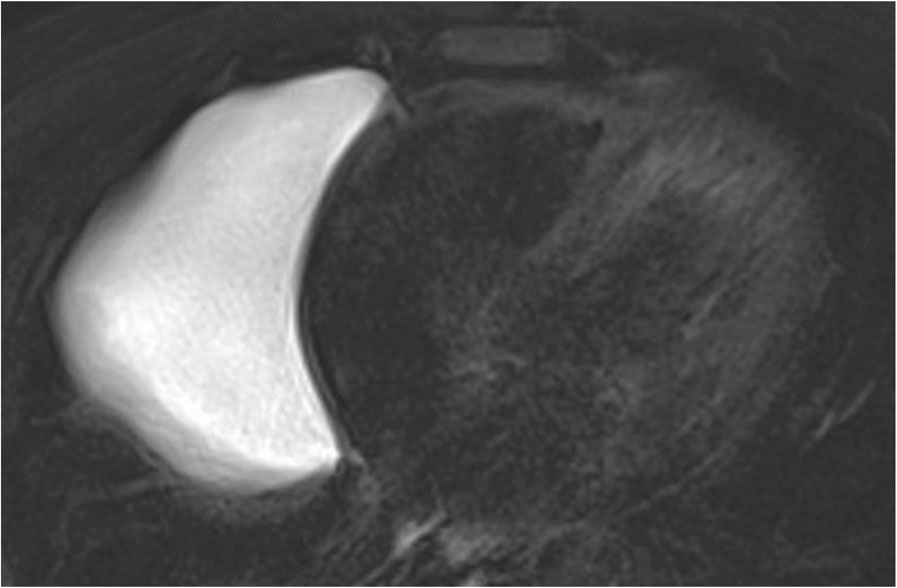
A 40-year-old man with incidentally detected pericardial cyst. Axial fat-suppressed T2-weighted MR image demonstrates hyperintense cystic mass in the right anterior cardiophrenic angle compatible with a pericardial cyst
